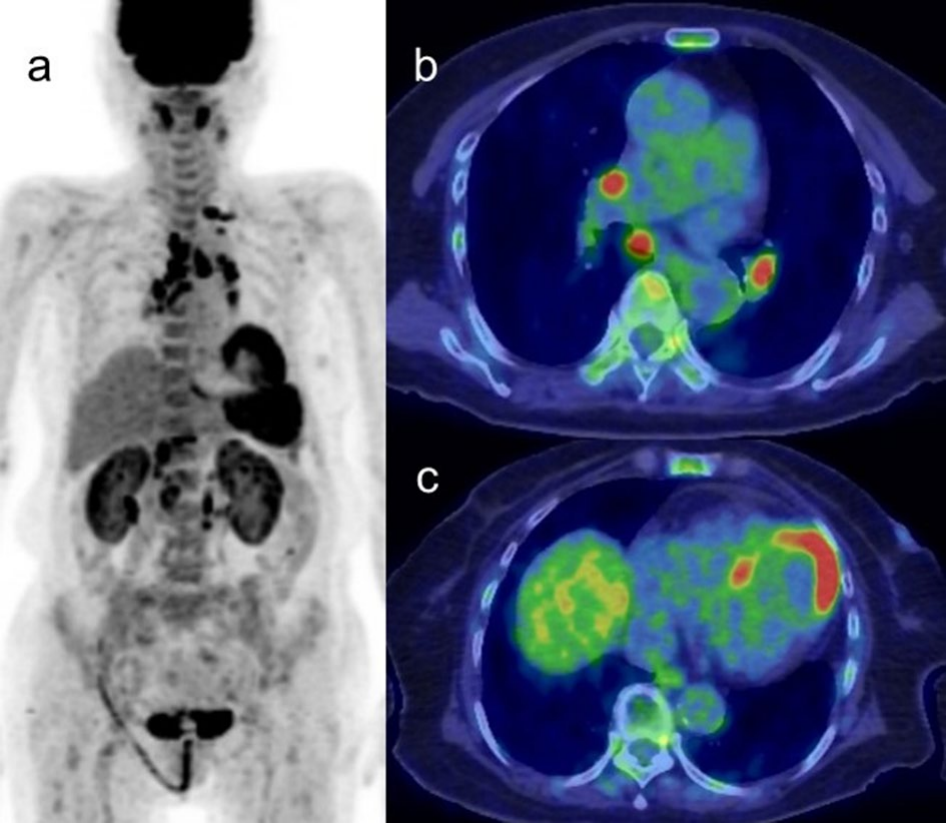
Cardiac sarcoidosis. Cardiac sarcoidosis in 70-year-old female. The patient showed multiple lymph node swelling on CT and suspected malignant lymphoma. FDG-PET/CT showed FDG uptake in the lymph nodes, and finally diagnosed with sarcoidosis. FDG-PET/CT presented intense FDG uptake in the left ventricular wall, finally diagnosed with cardiac involvement of sarcoidosis
